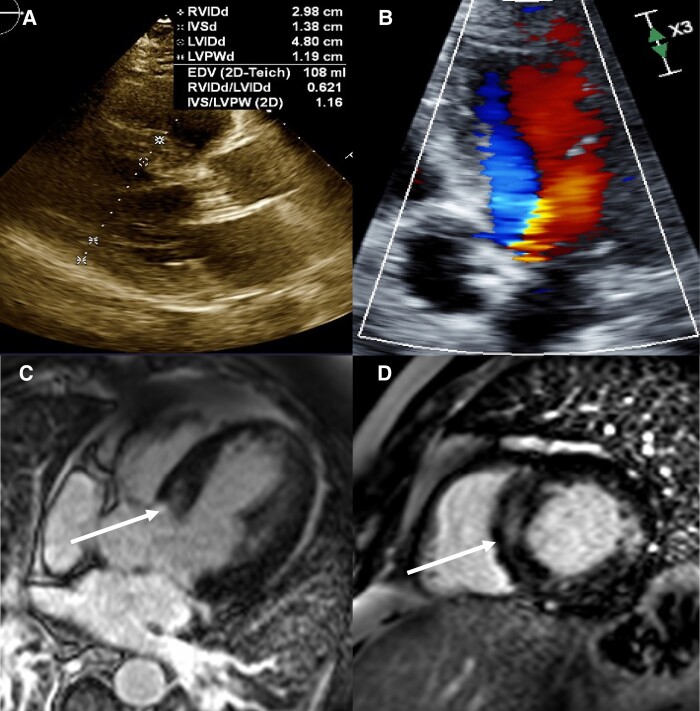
(*A*) Parasternal long transthoracic echocardiogram view likely underestimating intraventricular septal diameter. (*B*) Apical four-chamber transthoracic echocardiogram Doppler of left ventricular outflow tract obstruction. (*C*) Four-chamber cardiac magnetic resonance imaging view demonstrating intramyocardial late gadolinium enhancement (arrow). (*D*) Basal short-axis cardiac magnetic resonance imaging view of late gadolinium enhancement (arrow).
